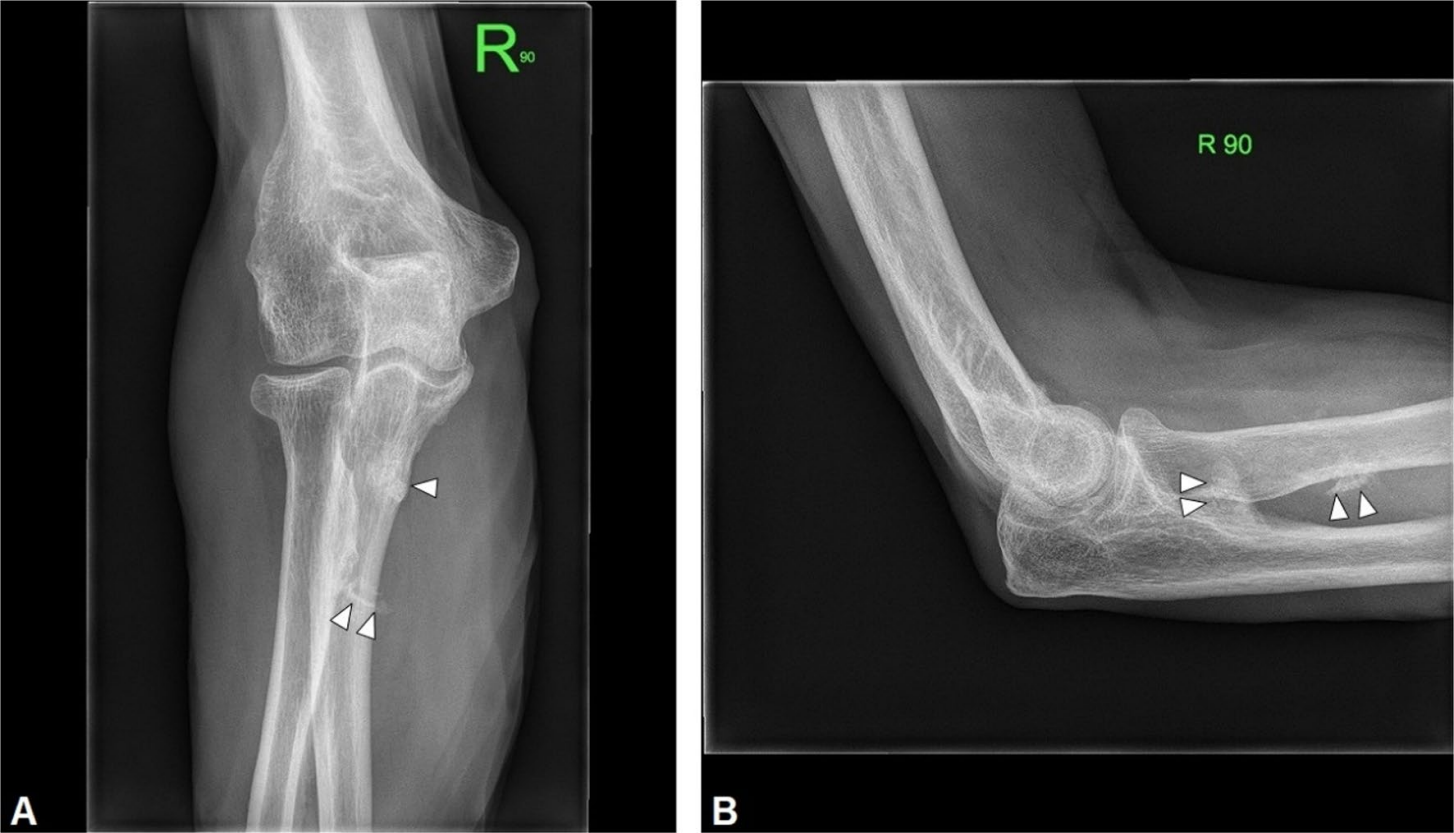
65-year-old man with a PMT of the right elbow. AP (A) and lateral (B) radiographs of the shoulder demonstrate an ill-defined area of soft tissue calcification in the proximal forearm
